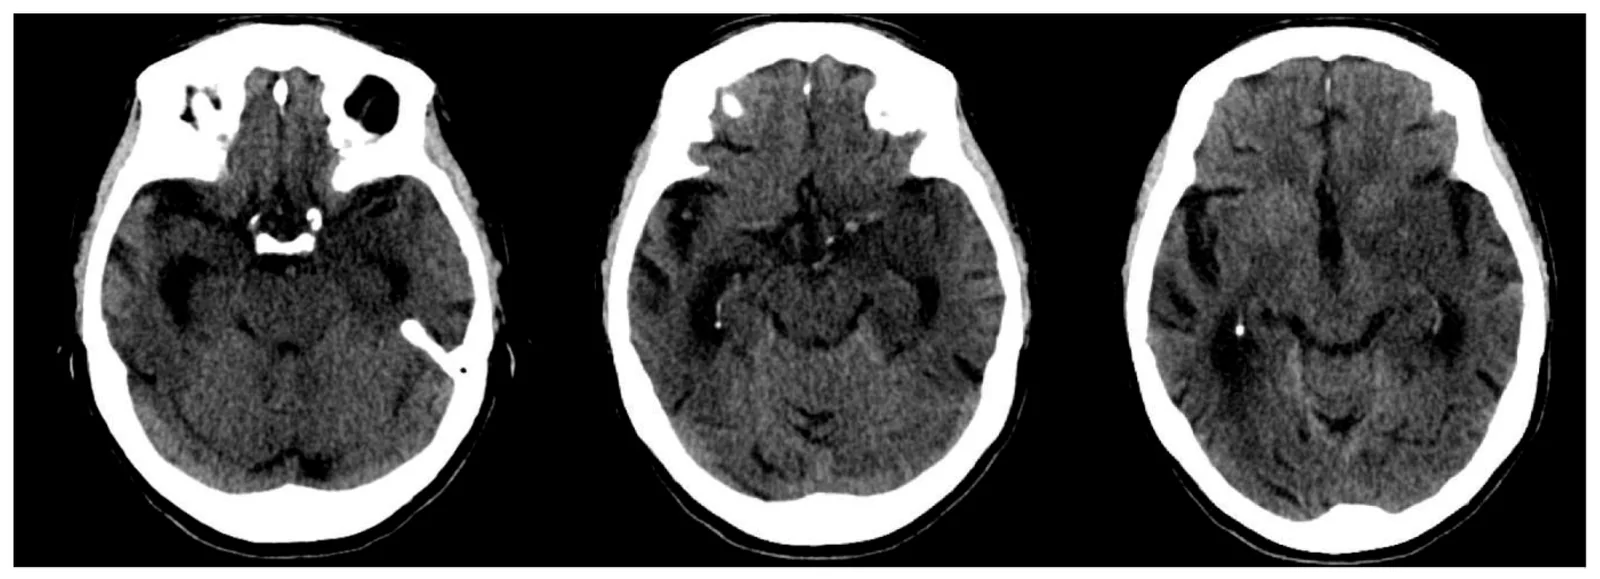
Head axial CT performed on 20 March 2026. A hypodense lesion in the left insular cortex with poorly defined margins, suggestive of a potential acute ischemic stroke, was observed.
